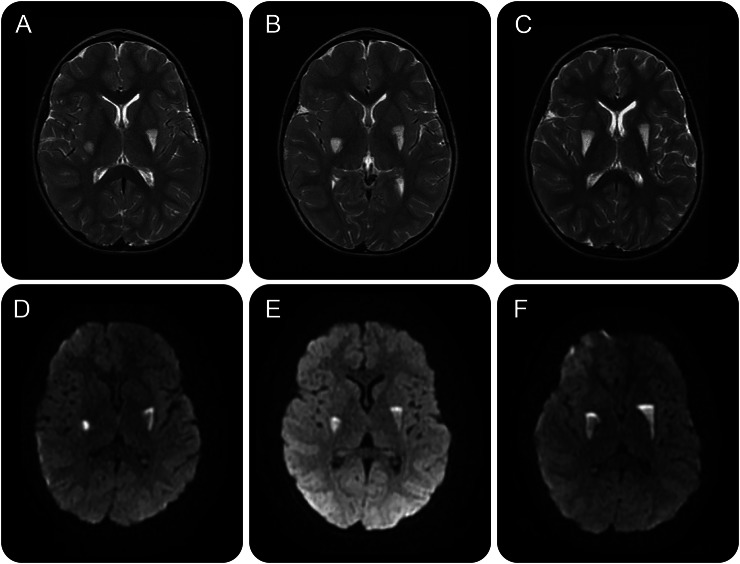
Serial MRI head images (T2 and diffusion-weighted) Serial T2 (A–C) and diffusion-weighted (D–F) images reveal an evolving hyperintense lesion in the right globus pallidus (age 4 years). This becomes symmetrical by age 5–6 years.
